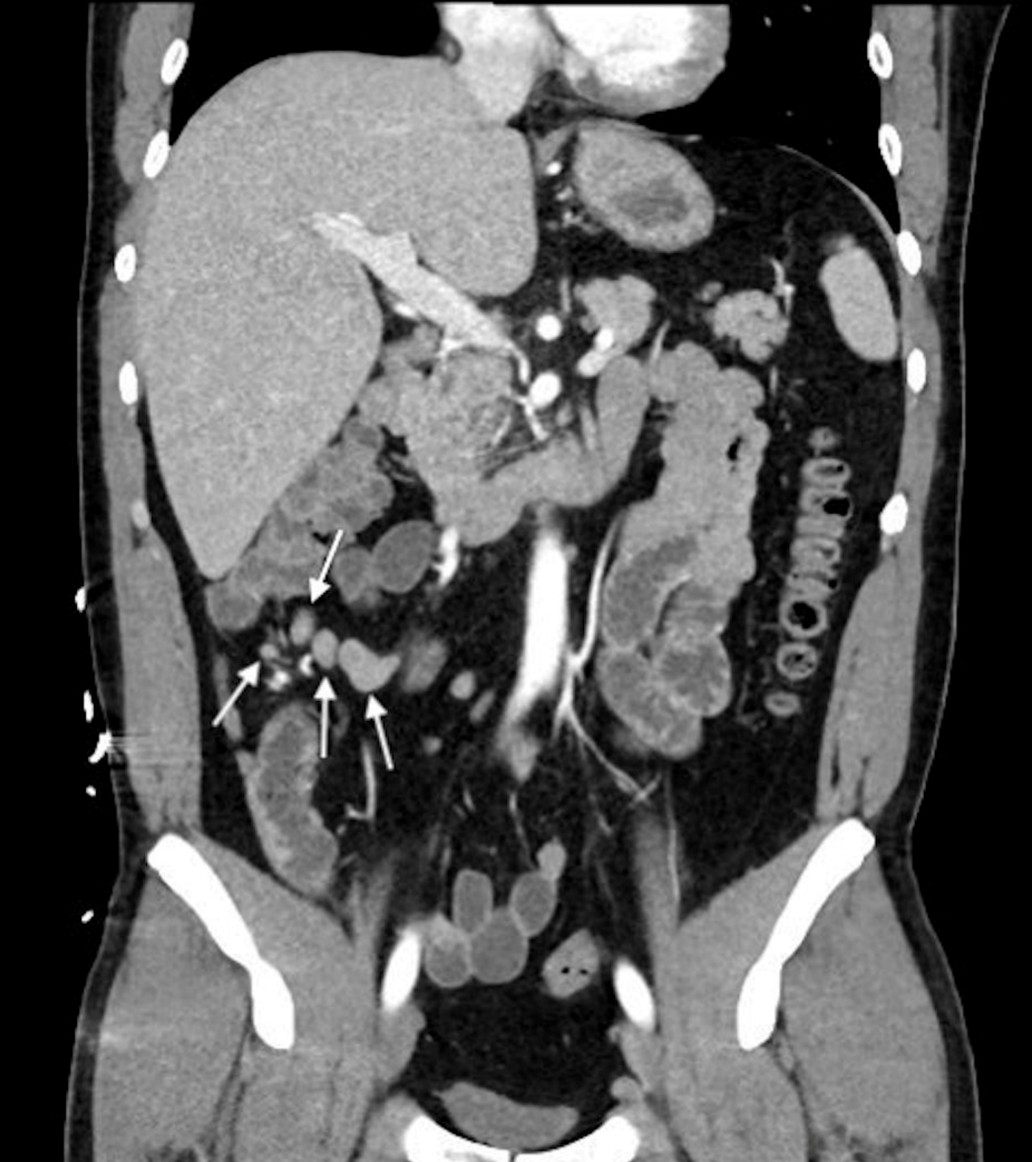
CT image of the abdomen with contrast revealing mesenteric adenitis (white arrows)
